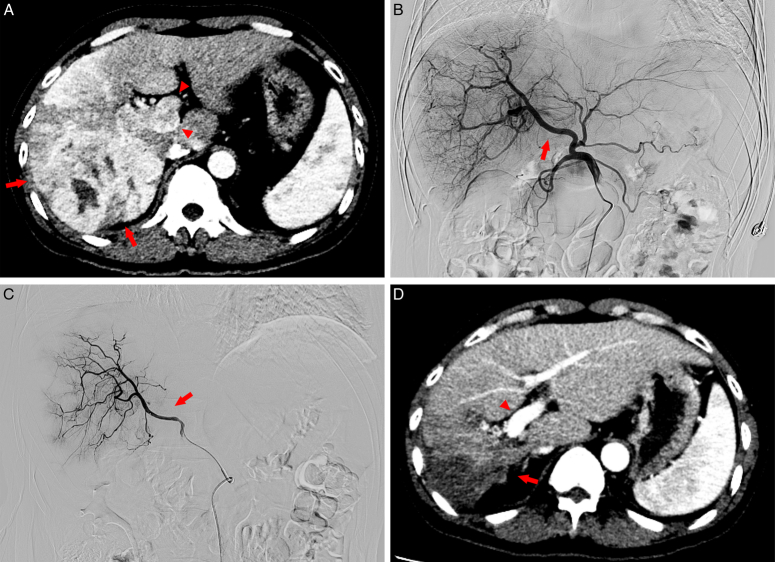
A 44-year-old man with huge hepatocellular carcinoma and Vp4 portal vein tumor thrombosis (PVTT) who received lenvatinib plus drug-eluting bead transarterial chemoembolization (DEB-TACE) and hepatic arterial infusion chemotherapy (HAIC). (A) Pretreatment contrast-enhanced computed tomography (CT) demonstrated an intrahepatic mass of 11.8 cm in the right lobe (arrows) with Vp4 PVTT (arrowheads). (B) Digital-subtraction angiography (DSA) before embolization showed the tumor-feeding vessels derived from right hepatic artery (arrow). (C) DSA after the first DEB-TACE showed that the tumor blush remained but significantly reduced, and the microcatheter was reserved at the right hepatic artery for HAIC (arrow); (D) Contrast-enhanced CT image obtained 2 months after three DEB-TACE and HAIC treatments demonstrated remarkable tumor shrinkage without enhancement inside the intrahepatic tumor and PVTT (arrow), with patency of the main trunk and left branches of portal vein (arrowheads).
